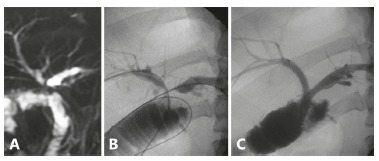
MRI of a hepaticojejunostomy stricture involving both hepatic ducts: A)
